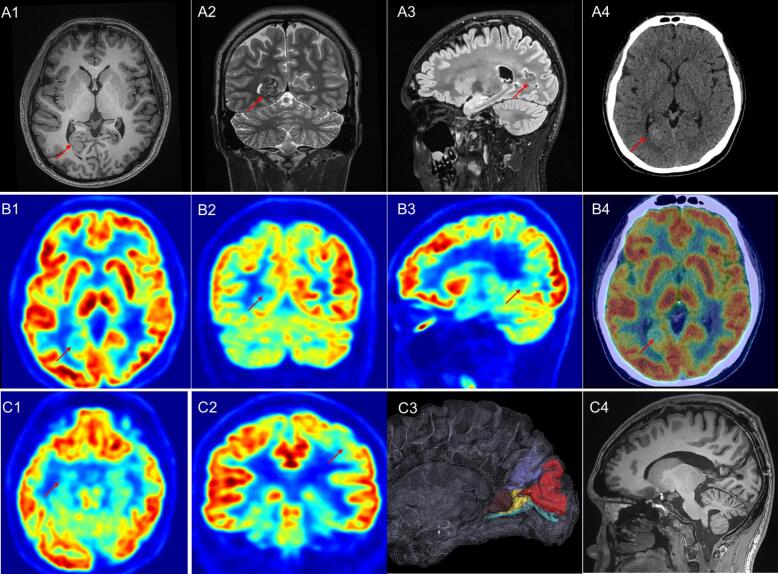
Structural neuroimaging and metabolic imaging. (A1-A4) Structural neuroimaging. (A1) Axial T1-weighted MRI shows cortical thickening with iso- to hypointense signal in the right cuneus (arrow). (A2) Coronal T2-weighted MRI demonstrates mixed iso- to hypointense signal within the lesion (arrow). (A3) Sagittal FLAIR MRI shows mixed signal abnormality (arrow). (A4) Axial CT revealed a focal hyperdensity consistent with calcification. (B1-B4). Lesion co-localization on FDG-PET. (B1) Axial, (B2) coronal, and (B3) sagittal FDG-PET images demonstrate focal hypometabolism in the right cuneus (red arrows), co-localizing with the structural lesion. (B4) Axial CT–PET fusion provides anatomical reference. (C1–C4) Remote metabolic involvement and postoperative outcome. (C1) Axial FDG-PET shows right mesial temporal hypometabolism (arrow). (C2) Axial FDG-PET shows left central hypometabolism (arrow). (C3) 3D surface reconstruction (Slicer/Freesurfer) depicts the anatomical context of the lesion: right cuneus (red), ventral posterior cingulate (brown), calcarine sulcus (blue), parieto-occipital sulcus (purple). The manually delineated lesion is shown in yellow. (C4) Postoperative (3-month) T1-weighted MRI confirms complete resection of the lesion. Abbreviations: CT, computed tomography. FDG-PET, Fluorodeoxyglucose positron emission tomography, MRI, magnetic resonance imaging
